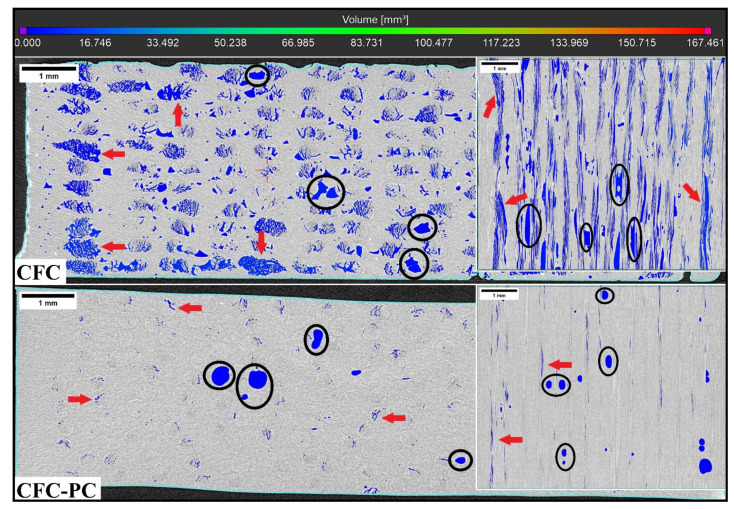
Top midsection CT images (X-Y) of the N-PLA with corresponding side midsection inset views (X-Z). A black circle is indicative of voids due to the thermoplastic matrix and red arrows showed voids within the CFC processed CCF.
